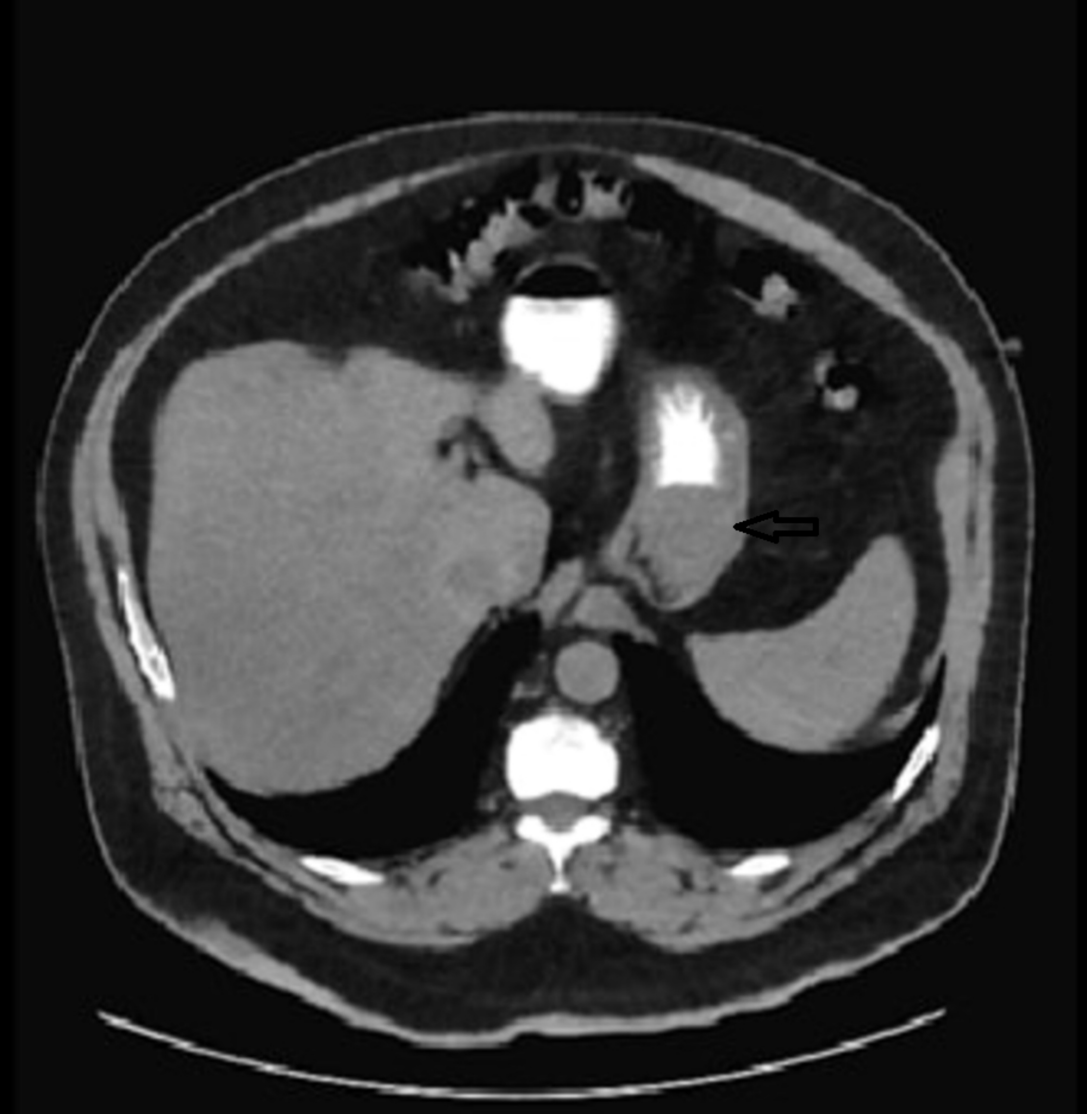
CT scan of the abdomen - transverse view showing a suspected mass in the stomach CT: computed tomography
